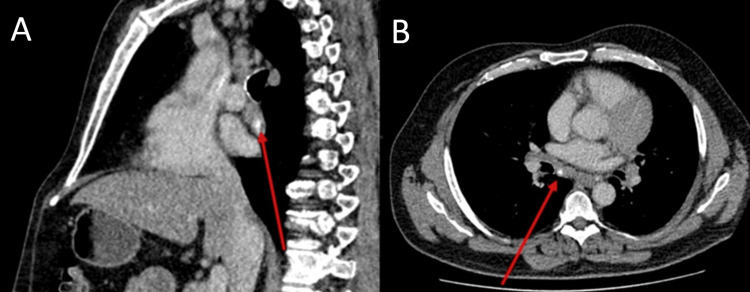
Contrast-enhanced CT thorax Sagittal (A) and axial (B) images demonstrating calcification within an enlarged subcarinal lymph node, highlighted by the red arrow.
